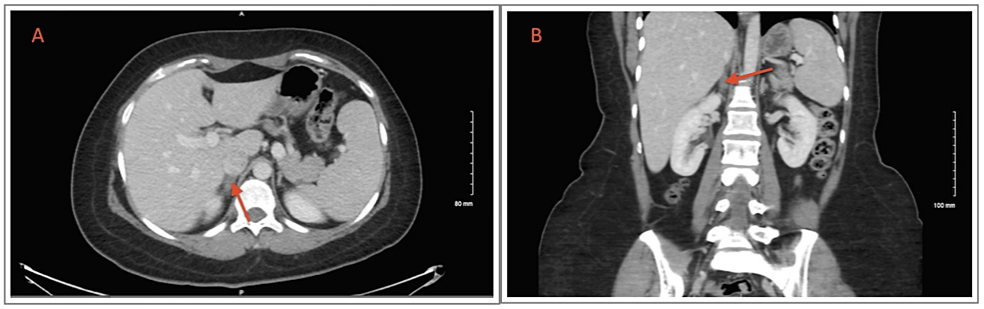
Axial (A) and coronal (B) views of CT scan demonstrate interval resolution of the previously seen right adrenal mass (arrows).
